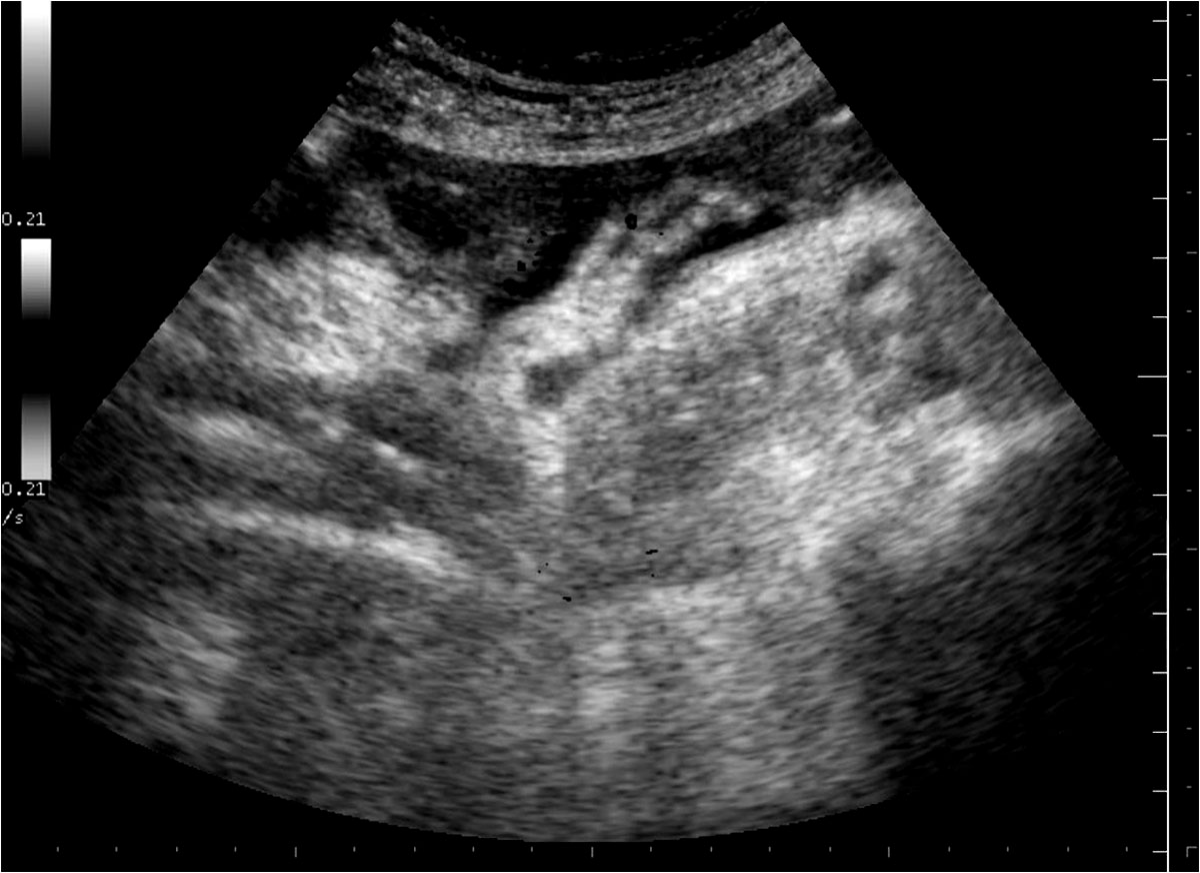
Direct sign, such localized gas collections related to bowel perforations, may be detectable, particularly if they are associated with other sonographic abnormalities, called indirect signs (thickened bowel loop and air bubbles in ascitic fluid or in a localized fluid collection, bowel or gallbladder thickened wall associated with decreased bowel motility or ileus)
